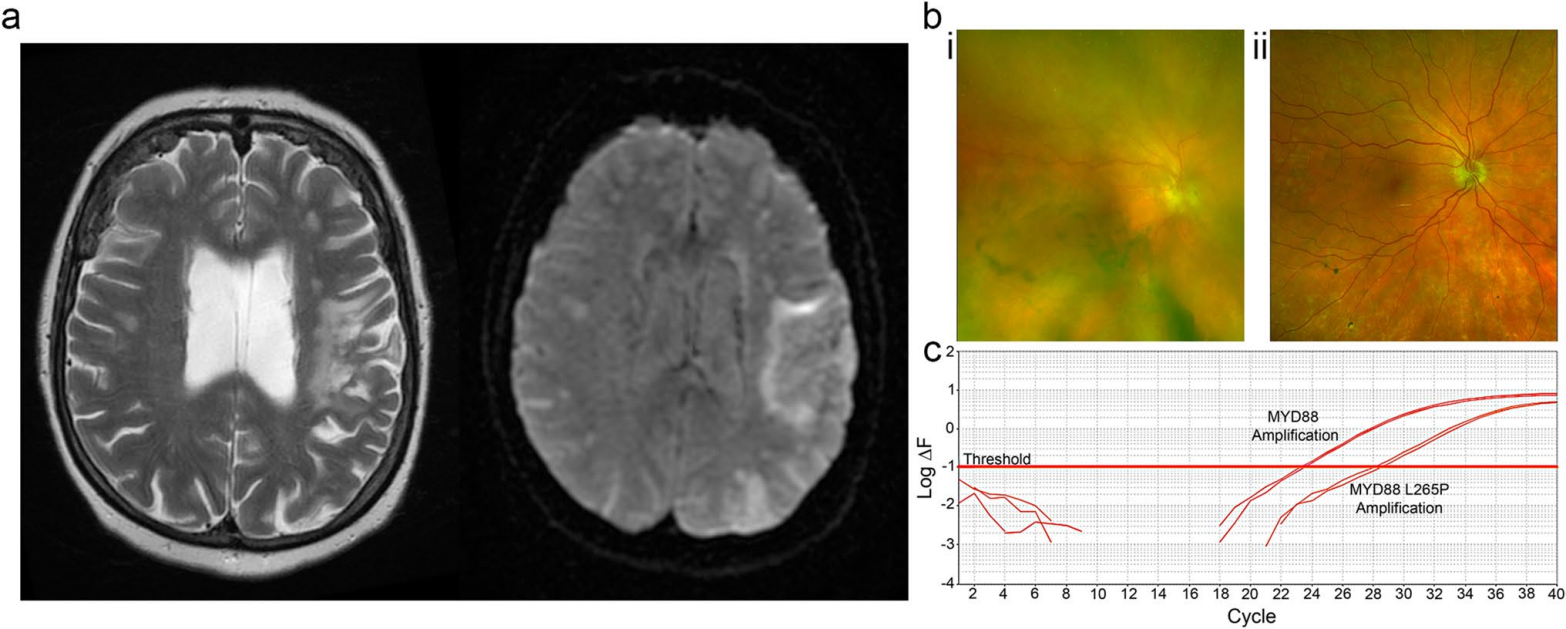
Diagnosis of primary CNS lymphoma with vitreous biopsy. a Patient presented with right-sided weakness with stuttering progression over several months. Left panel, T2-weighted MRI, increased signal in the territory of the left middle cerebral artery. Right panel, Diffusion-weighted imaging, increased signal suggesting restricted diffusion in left-hemisphere white matter. b Photographs of right fundus for patient in a: (i) before vitreous biopsy, retina obscured by cloudy vitreous; (ii) post-vitreous biopsy, retina visible, no sub-retinal deposits identified. c Detection of MYD88 L265P mutation by allele-specific PCR. The test compares the patient’s DNA with a positive control that has MYD88 L265P at 0.625%. The test relies on a failure of extension of the primers when there is mismatch between the primer and the extracted DNA. Any difference between the patient’s sample and the positive control is amplified by repeated PCR cycles and is measured (Δ*F*). The presence of the MYD88 L265P mutation is reported when the difference crosses a threshold
